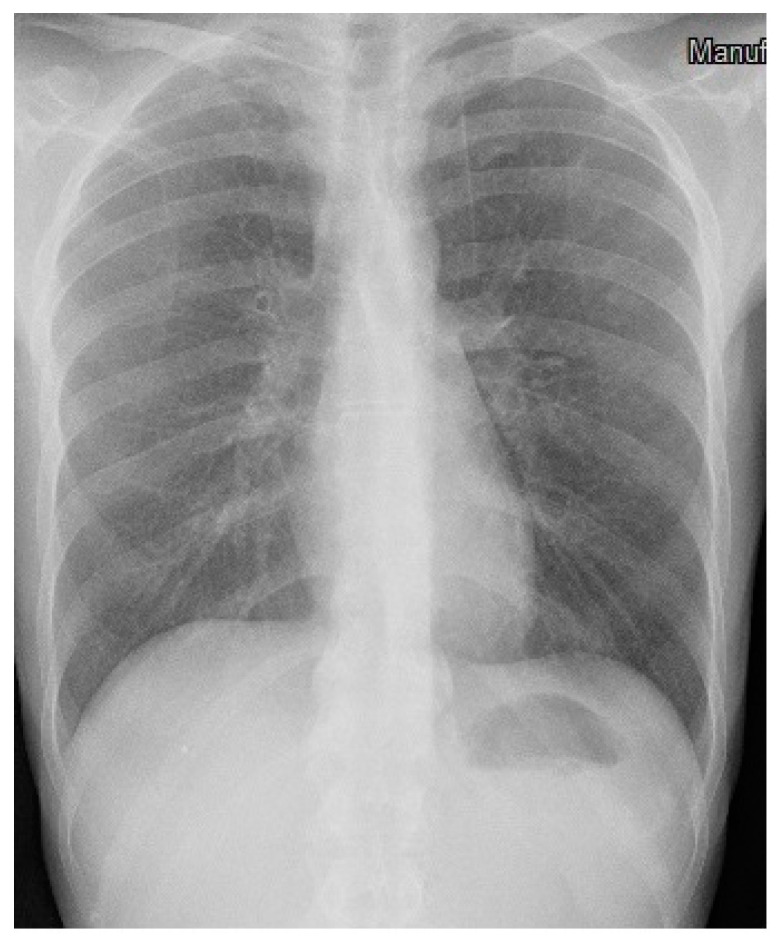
Follow-up chest X-ray of case n. 1.
